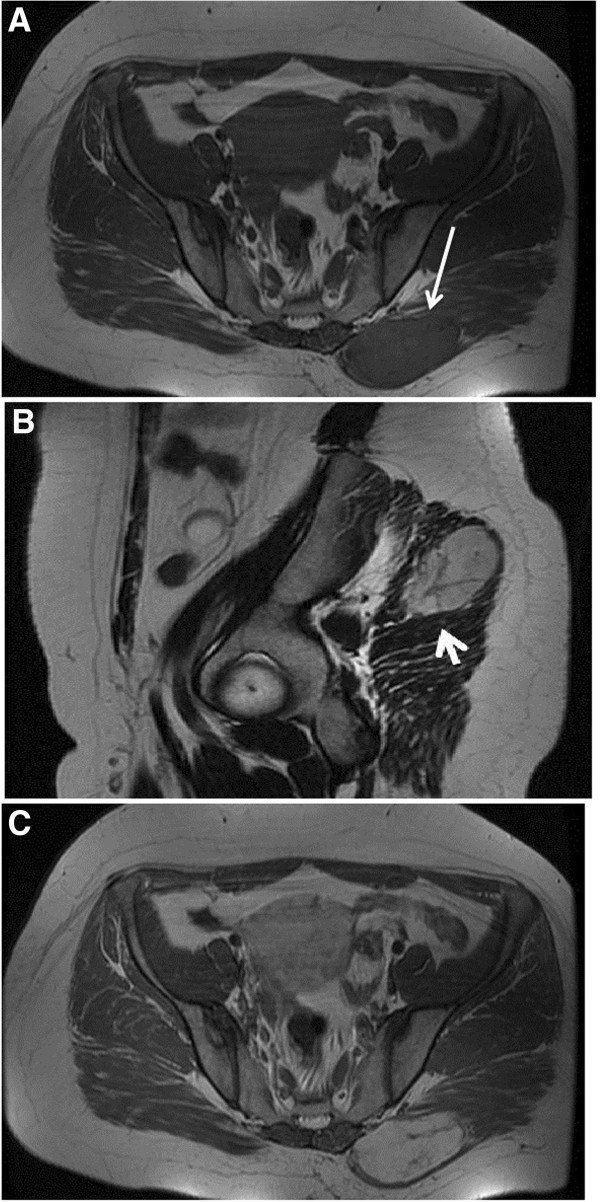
A deep soft tissue mass is intramuscularly located at the right gluteus maximus muscle (white arrow). The mass is isointense relative to adjacent muscle on T1 MR images (A). The mass has high SI and contains septations on T2MR image (B: white arrowhead). On gadolinium –enhanced T1 images (C), the lesion displays marked homogeneous enhancement. Internal septations remain unenhanced.
